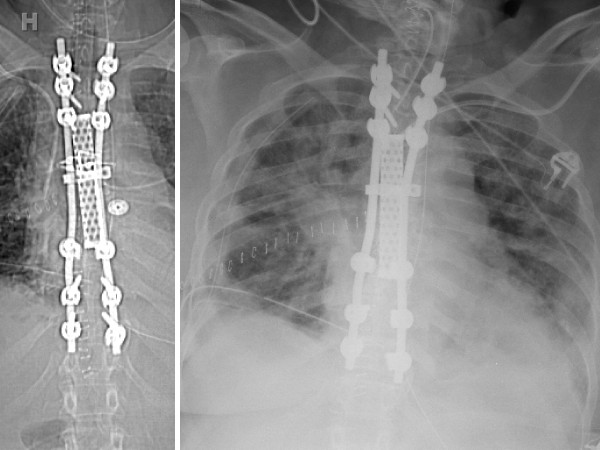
Postoperative CT scout (A) and chest X-ray (B) after 360° revision fixation by posterior instrumentation T2–T11 and anterior PMMA/Tobramycin mesh cage placement.
